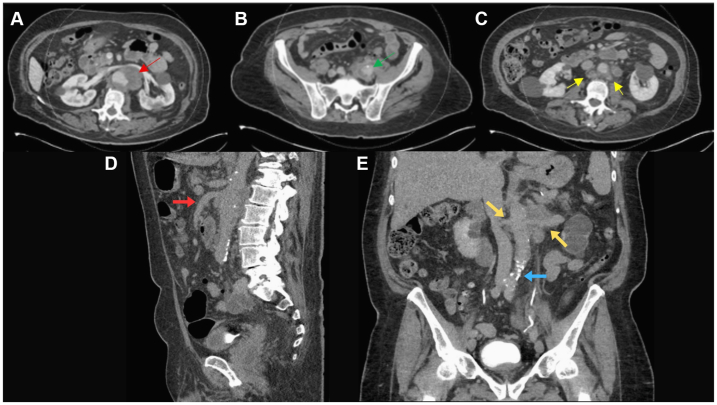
Representative axial images from computed tomography (CT) scans with intravenous (IV) contrast on initial presentation that demonstrated a broad-based outpouching of the suprarenal abdominal aorta measuring 1.3 × 1.4 × 2.1 cm, indicated by the *red arrow*(A) and a broad-based outpouching from the distal common iliac artery measuring 0.9 × 1.3 × 1.8 cm, indicated by the *green arrow*(B). CT imaging also demonstrated significant necrotic lymphadenopathy of the para-aortic lymph nodes (C) as well as left iliac chain lymph nodes, indicated by the *yellow arrows*. Coronal images (D) demonstrating a broad-based outpouching of the suprarenal abdominal aorta with superior mesenteric artery (SMA) involvement, indicated by the red arrow. CT imaging also demonstrated renal arteries surrounded by dense lymphadenopathy (*yellow arrow*) on a background of enlarged lymph nodes and a narrow bifurcation, indicated by the *blue arrow*(E).
